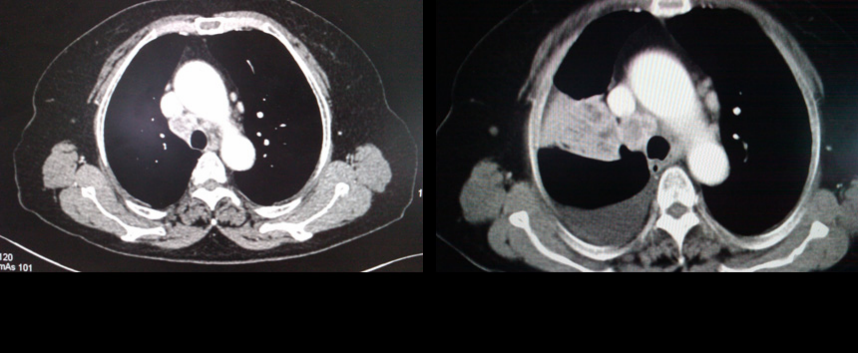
Case 3 showed volume increase of primary tumor and obvious hydrothorax after gefitinib therapy, as determined by thorax CT scan.
